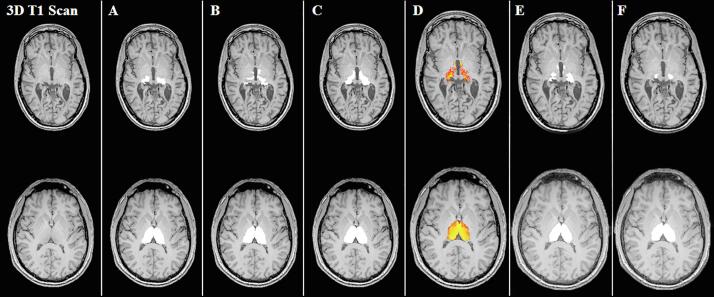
3D T1-weighted images and thalamus segmentations of manual tracing, FreeSurfer, FSL-FIRST, CAT12, GIF and VolBrain. Segmentations of the thalamus bilaterally in the axial plane of two MS patients, revealing the inferior portion of the thalamus of one cognitively impaired patient (top row) and the middle part of the thalamus of one cognitively preserved patient (bottom row) for: manual tracings (A), FreeSurfer (B), FSL-FIRST (C), CAT12 (D), GIF (E) and VolBrain (F) segmentations.
